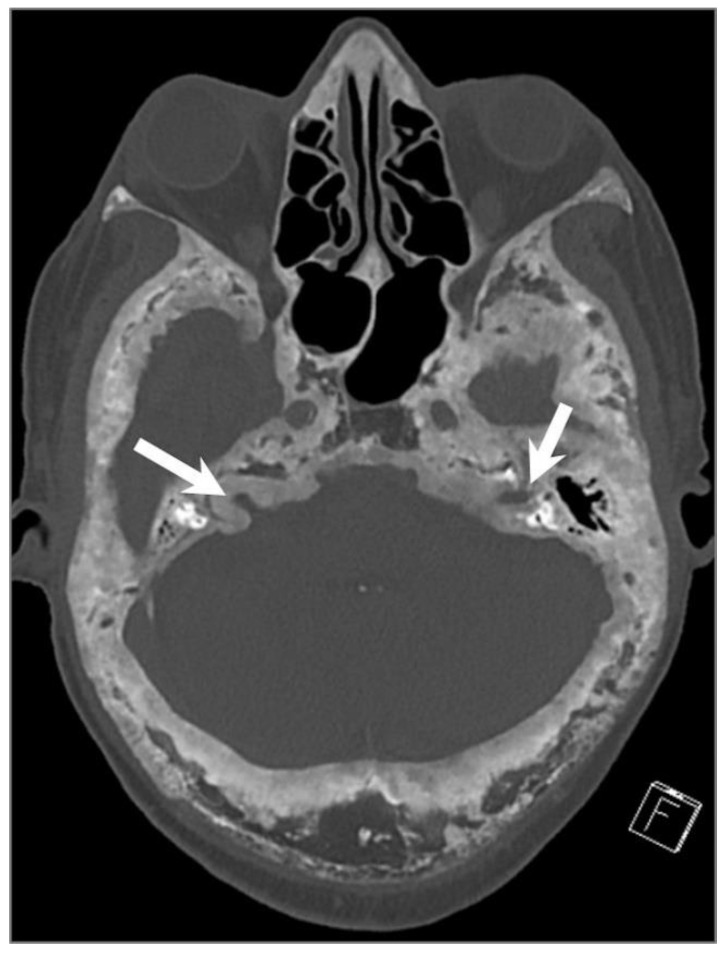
Paget’s disease. Axial CT image shows characteristic mixed lytic and sclerotic areas (‘cotton wool’ appearance) with thickened trabeculae, bone expansion, cortical thickening, and deformity of the calvarium and skull base. There is narrowing of the bilateral internal auditory canals due to cortical thickening (arrows).
